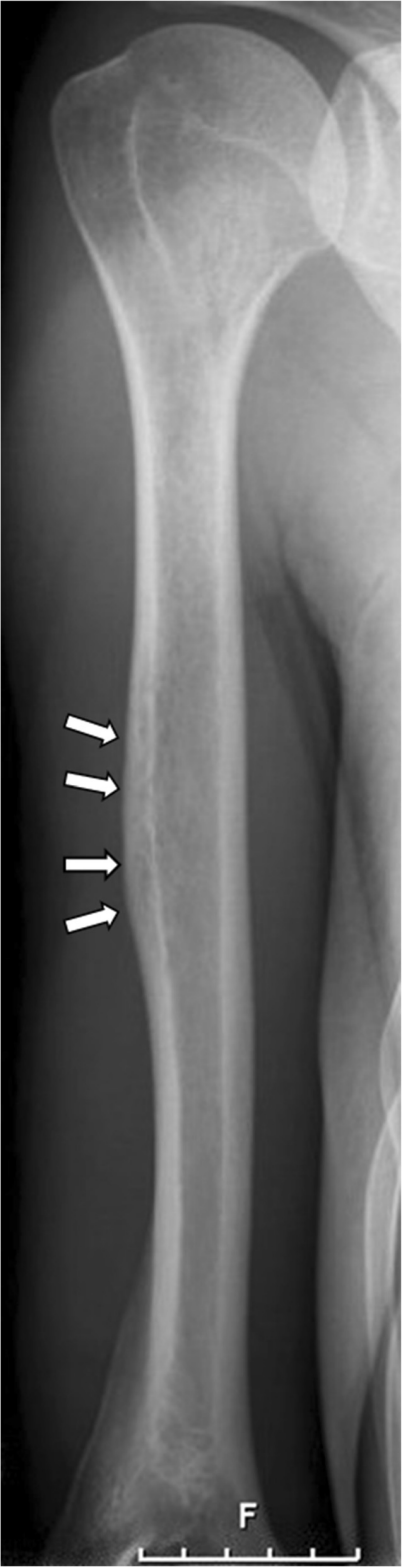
Radiograph of the right humerus: cortical disaggregation suggesting a permeative process which can be seen at the lateral, diaphyseal corticalis (arrows).
